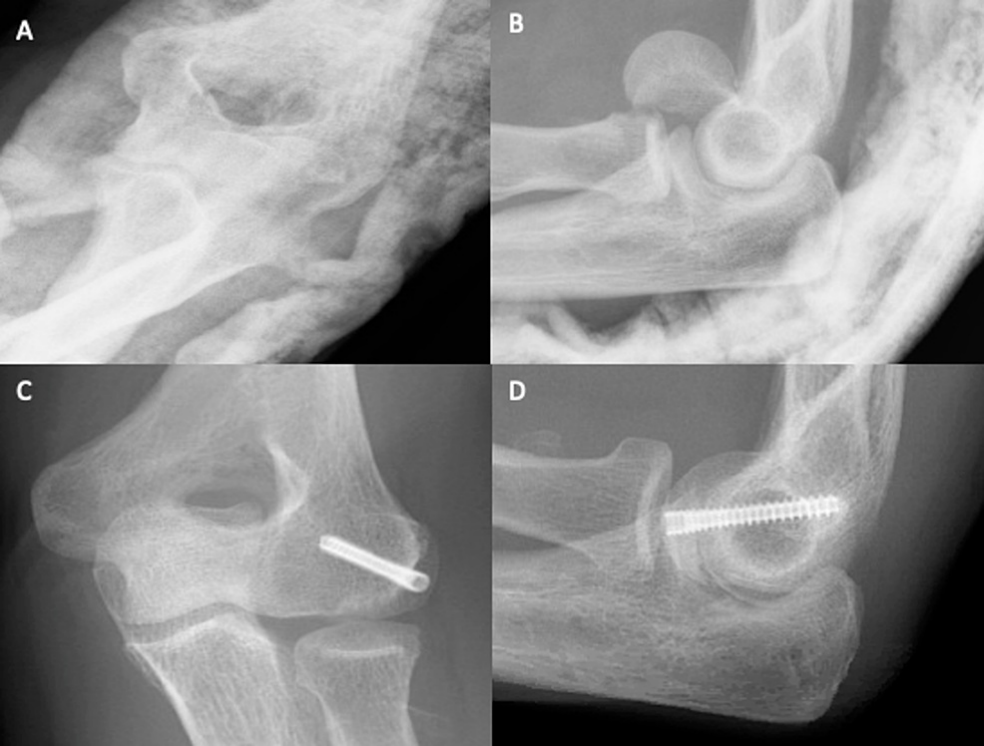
Plain elbow X-rays of case 7. Figure 9A, 9B shows anteroposterior and lateral views, respectively, of a type I capitellum fracture. The backslab applied in the emergency department can be seen. Figure 9C, 9D shows anteroposterior and lateral views, respectively, of the elbow after ORIF. ORIF: open reduction and internal fixation
